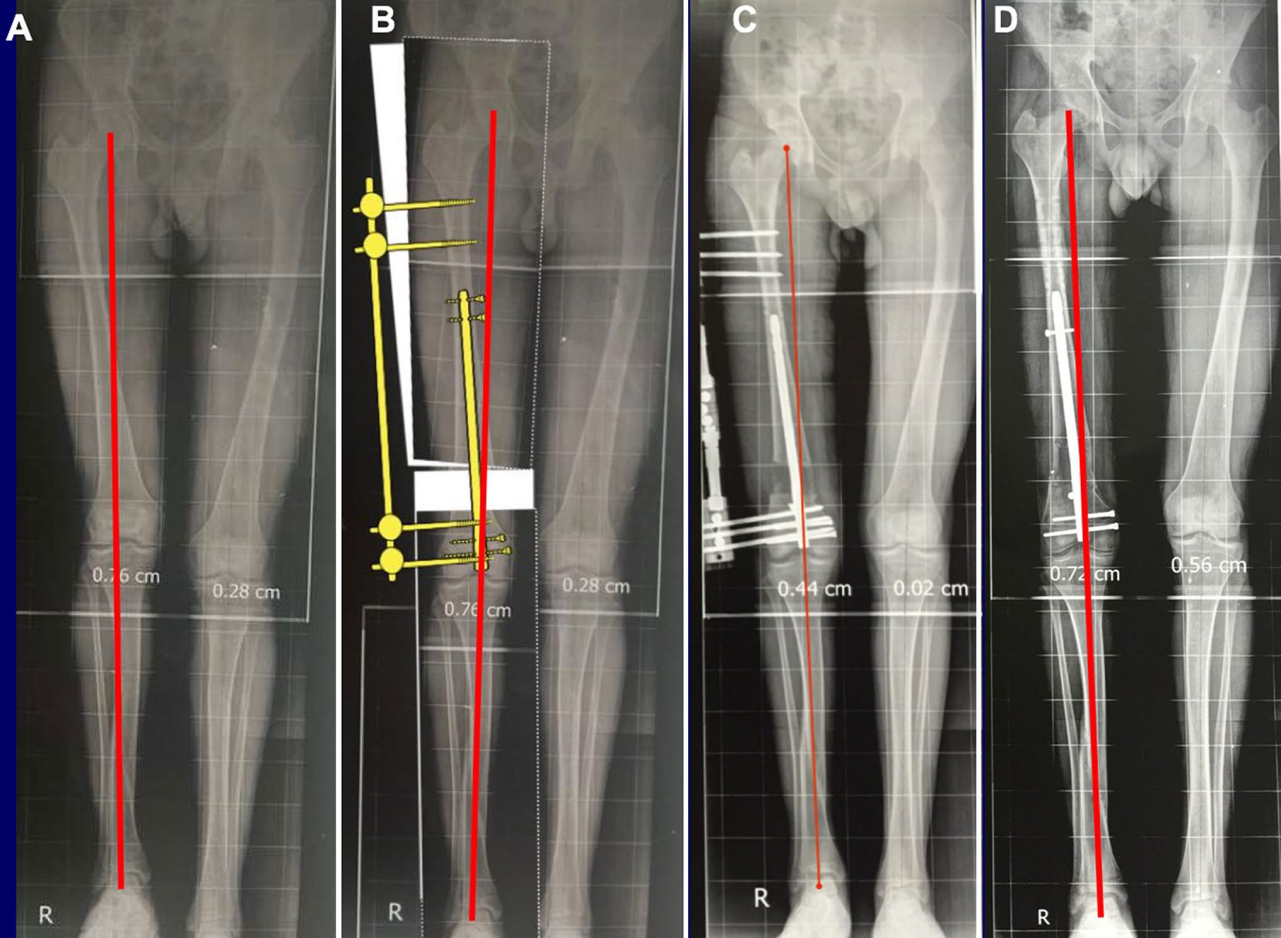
a Pre-operative radiograph with a slight lateral MAD, b the proposed planning to achieve normal limb alignment after lengthening is completed, c the reproduction of the planning and achieving normal alignment at the end of lengthening with no residual MAD, d the final radiograph after regenerate consolidation with normal limb alignment and no residual MAD
